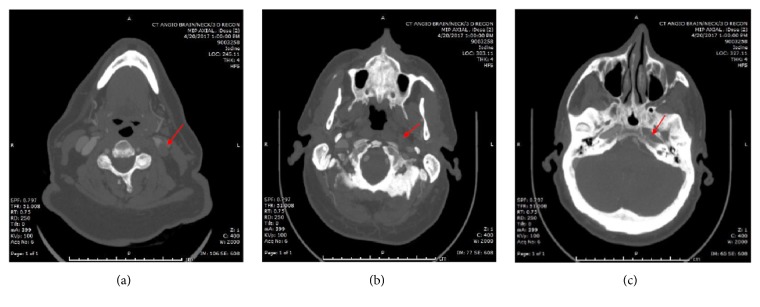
CT angiogram head and neck. Note the occlusion of the left internal carotid artery, starting at the level of the common carotid bifurcation (a), in the neck (b), and in the carotid canal (c).
